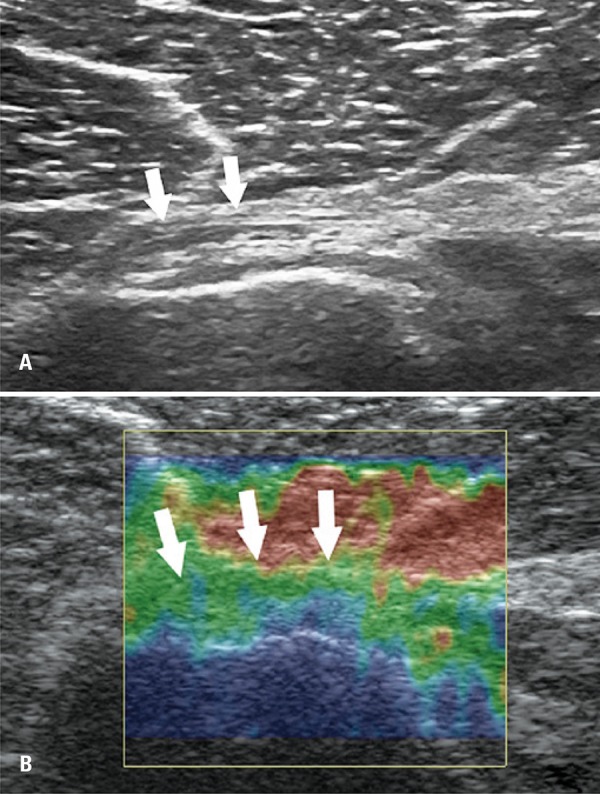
Ultrasound assessment of normal pectoralis major muscle tendon. (A) Normal tendon with fibrilar pattern and common echogenicity (white arrows). (B) Elastography assessment showing intermediate pattern (Green)
